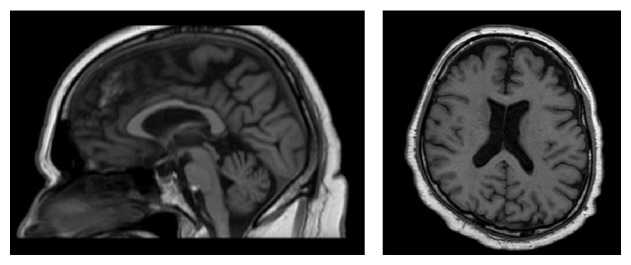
The MRI scan of the brain revealed significant cerebral atrophy.
